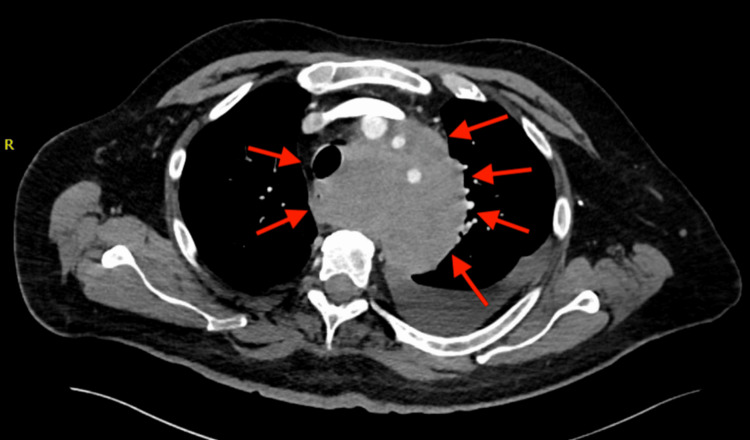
CT scan performed on July 31, 2024, revealing a newly discovered mediastinal mass (red arrows)
